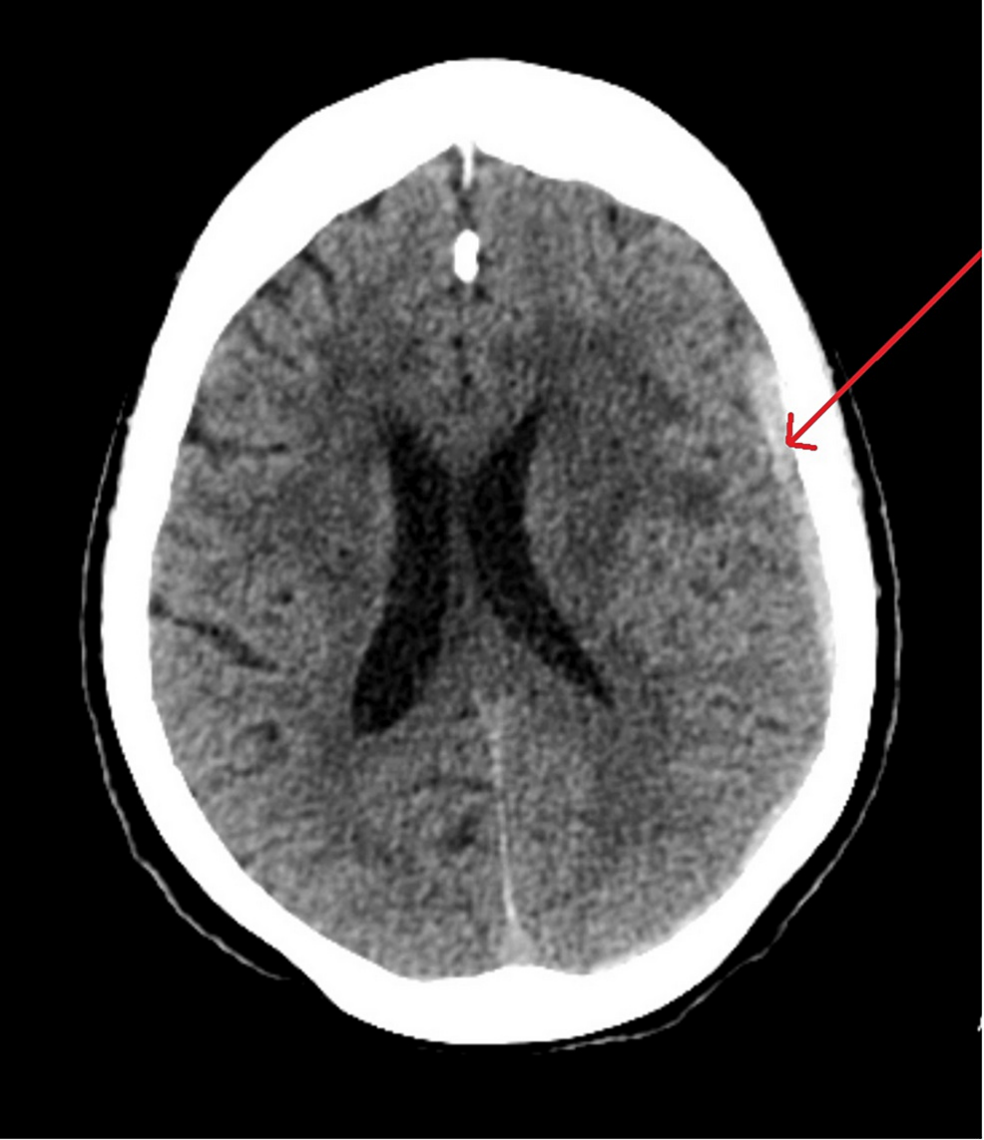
Axial view of computed tomography of the brain: acute subdural hematoma pointed by the red arrow.
